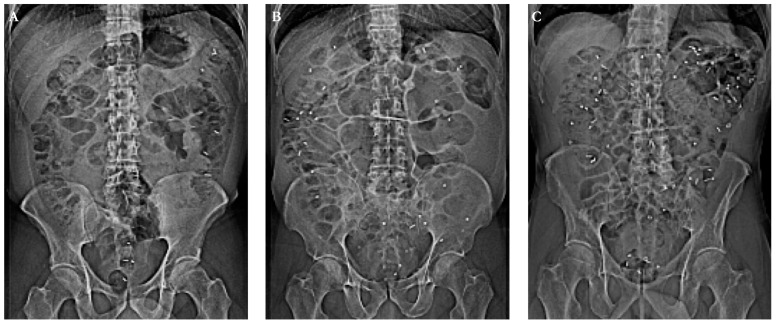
Abdominal x-ray topograms visualizing the retention of radio-opaque markers in the gastrointestinal tract as an objective measure of colonic transit time (CTT). (A). Healthy control with an estimated CTT within the normal range. (B). Parkinson’s disease (PD) patient with an estimated CTT near the mean for PD patients. (C). PD patient with severely prolonged CTT.
